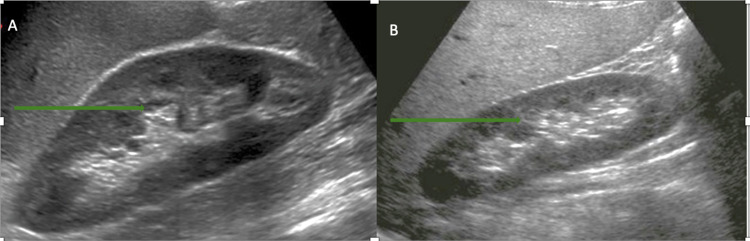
Ultrasound (sagittal view) of left kidney (A) and right kidney (B) demonstrating resolution of hydronephrosis.
